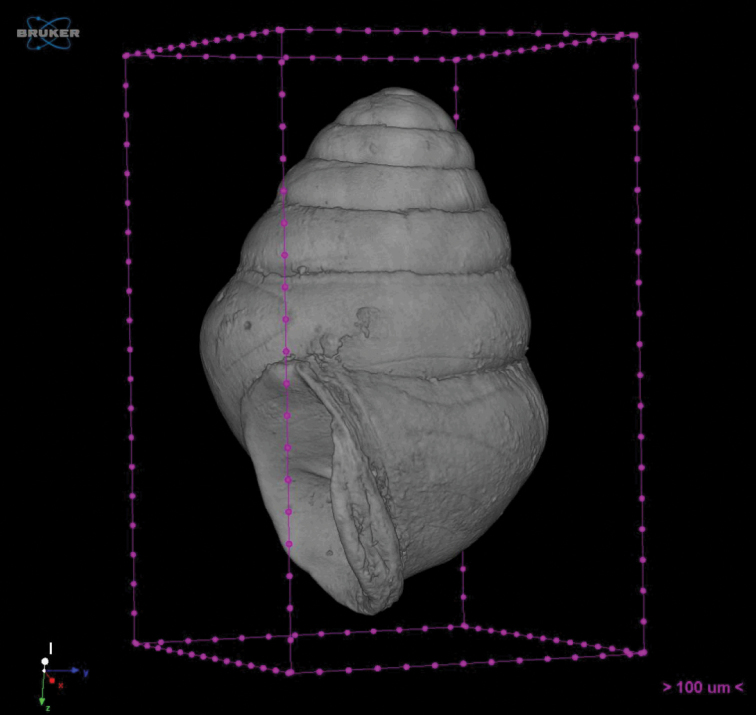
Nano-CT film of *Koreozospeum
